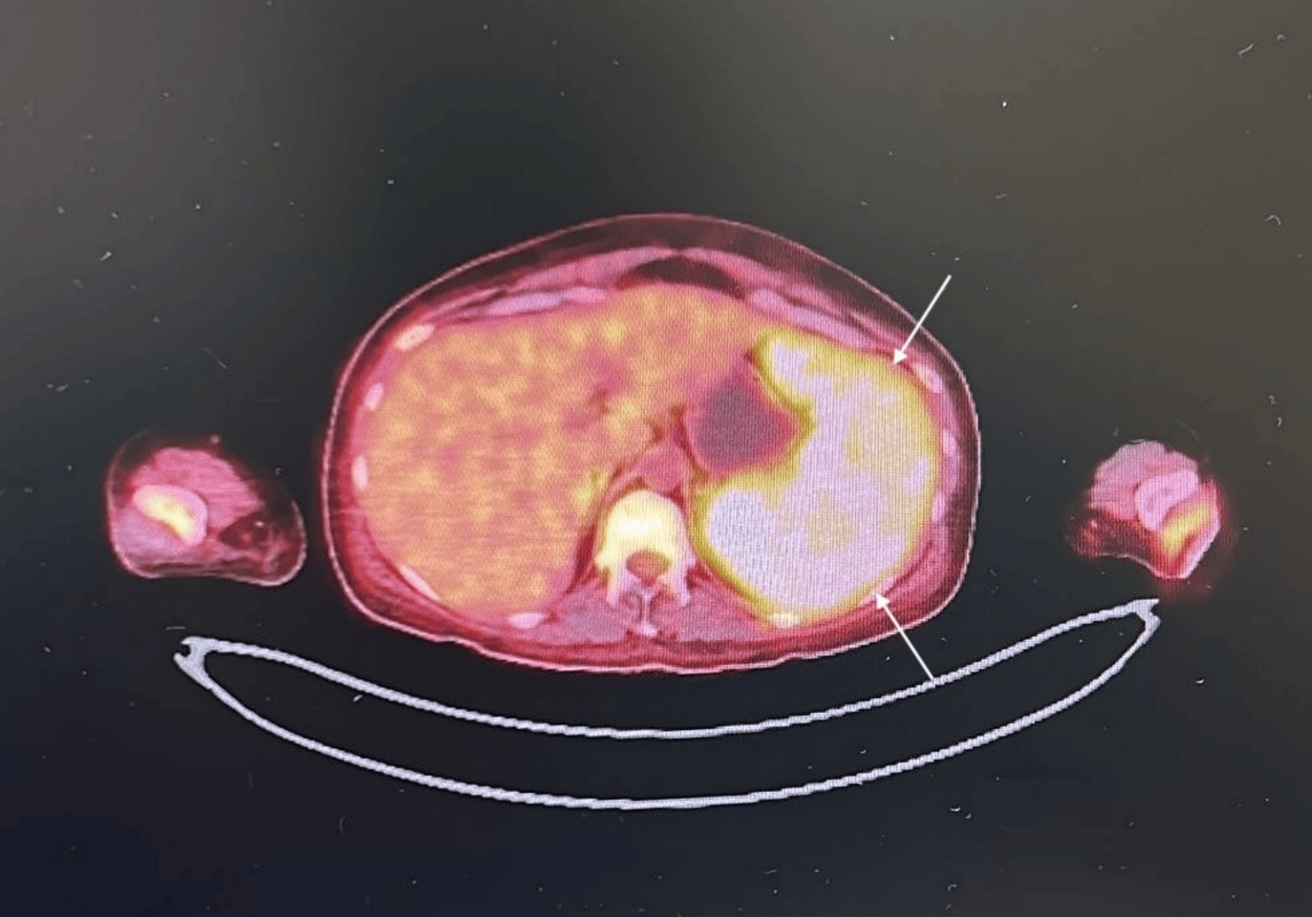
Diffuse splenic involvement in PET-CT. PET-CT: positron emission tomography-computed tomography
